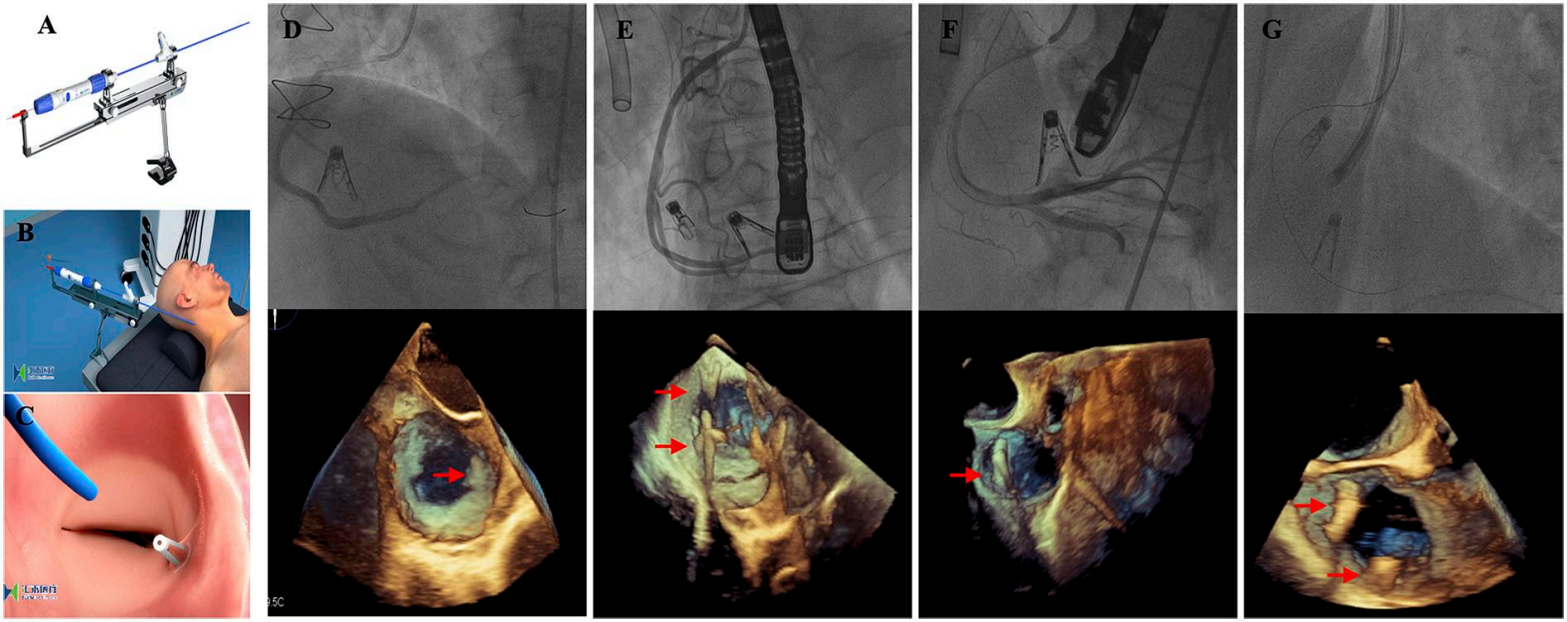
Procedural technique and results. (A) The K-Clip™ system; (B) The procedure was performed under general anesthesia and transjugular access; (C) After the clip released, the tricuspid annulus length was reduced; (D–G) The K-Clip™ device was successfully implanted in all four patients—2 patients with 2 clips each and 2 patients with 1 clip each, and 3D transesophageal echocardiography displayed the position of the clips (red arrow).
